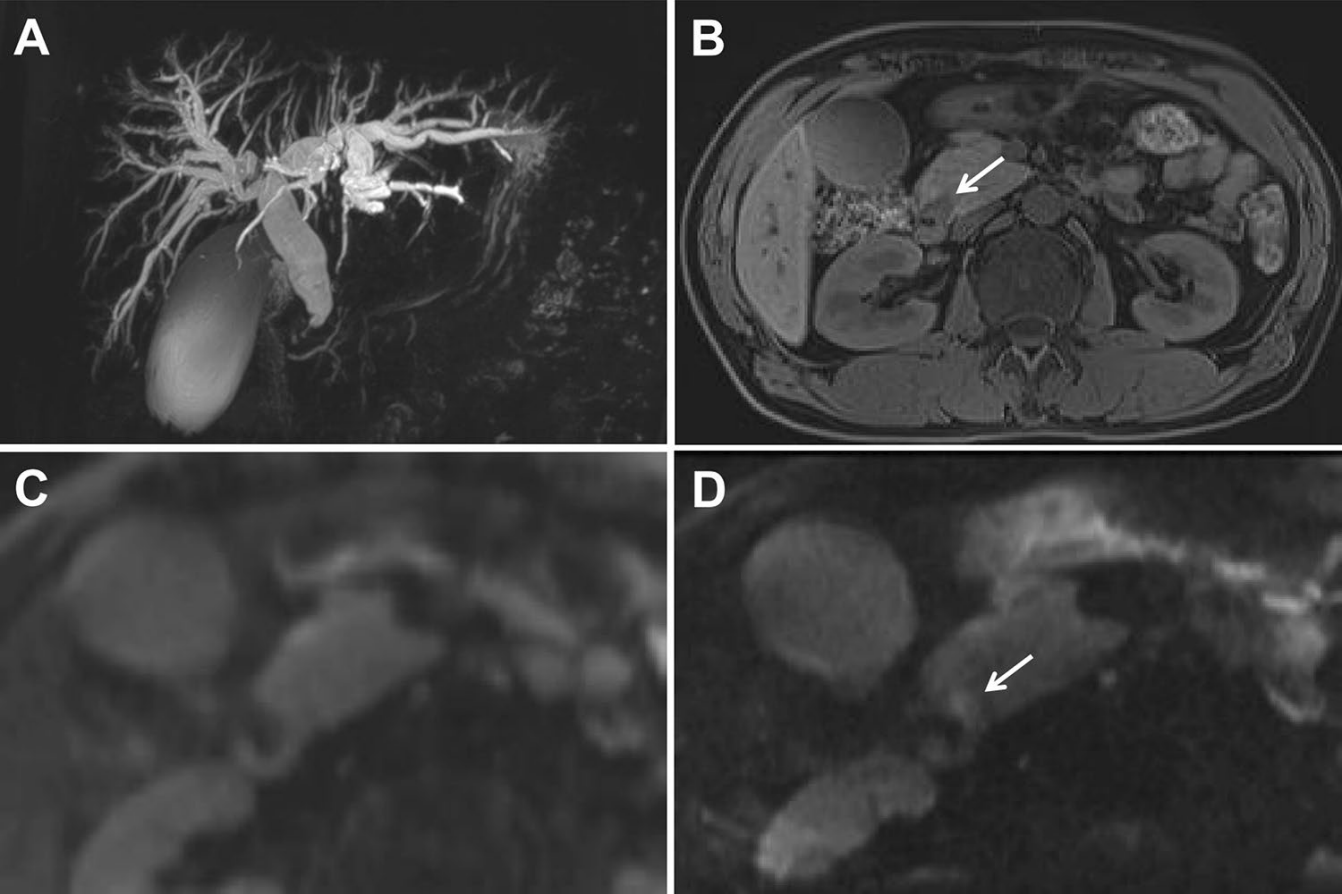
A 49-year-old man with distal common bile duct (CBD) carcinoma with a diameter of 9.9 mm. A MRCP shows marked bile duct dilatation with stenosis of the distal common bile duct (CBD) carcinoma. Main pancreatic duct dilatation was not noted. B T1-weighted MR image shows a hypointense nodule (arrow) in the periampullary region. C c-EPI DWI shows no abnormality in the periampullary region. D z-EPI DWI shows increased signal intensity of the lesion with better delineation
